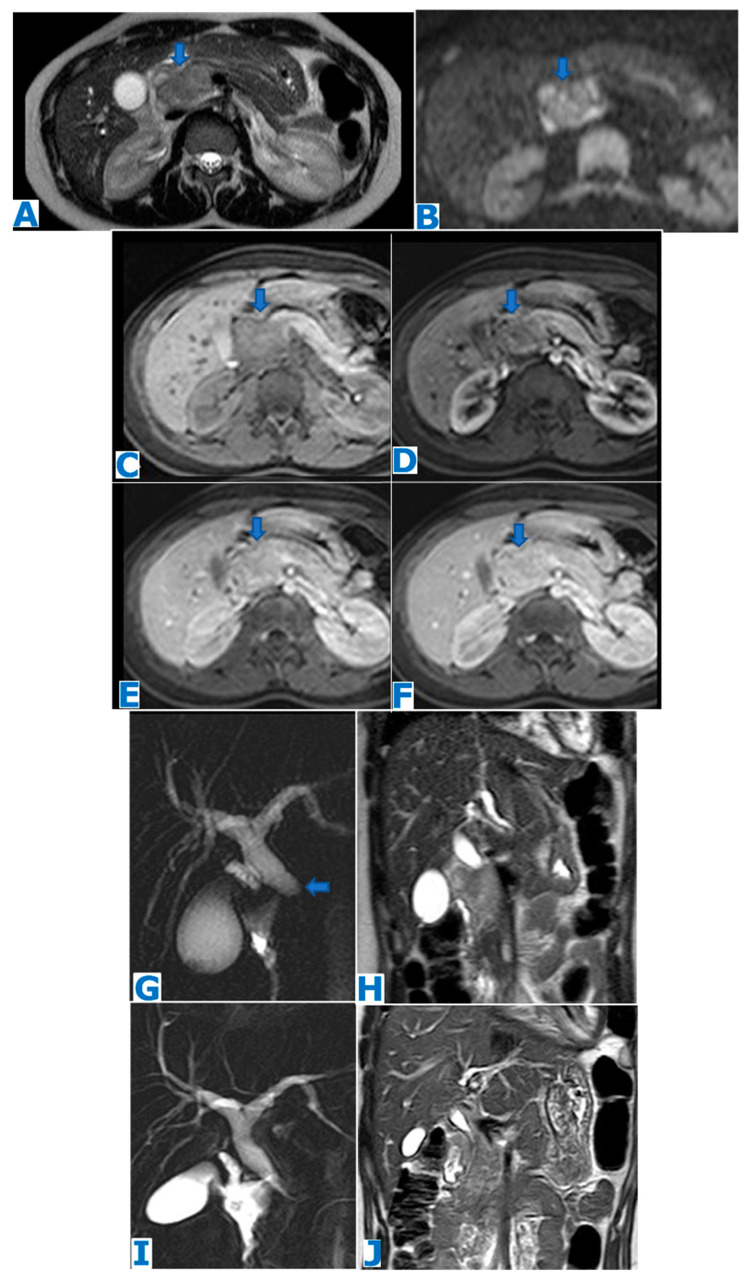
(A): axial T2WI, (B): DWI, (C): NCE FST1WI, (D–F): axial CE dynamic FST1WI (D): pancreatic parenchymal phase, (E): portal venous phase, (F): delayed venous phase, (G): MCRP, (H): coronal T2WI, (I): posttreatment MCRP, (J): posttreatment coronal T2WI. Primary pancreatic tuberculosis in a 15-year-old patient from Burundi presenting with abdominal pain and anicteric cholestasis. Note in the T2WI a hyperintense mass in the head of the pancreas (arrow in (A)) causing an abrupt biliary duct cutoff (arrow in (G)) and upstream dilatation. The mass shows diffusion restriction (arrow in (B)) and progressive enhancement in the dynamic sequences (arrows in (C–F)). EUS-guided FNA revealed necrosis, Langhans giant cells, lymphocytes and macrophages organised in granulomas. Thoracic radiography (not shown) was normal, and diagnosis was primary pancreatic tuberculosis. Both the lesion and mass effect on the common bile duct completely resolved after treatment (I,J).
